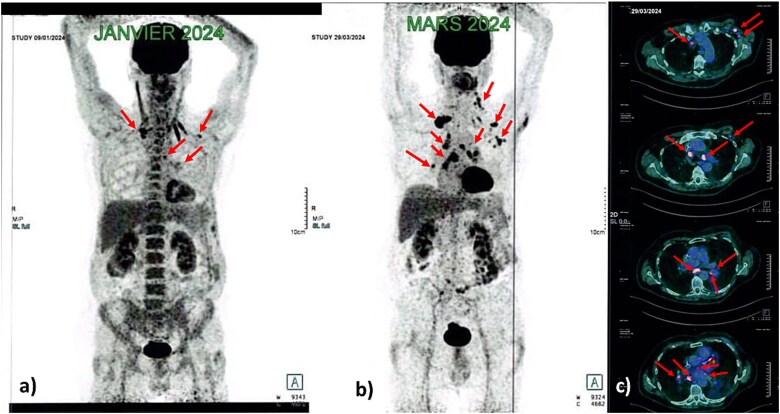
MIP view of FDG PET/CT from January 2024 (a) and march 2024 (b) showing metabolic progression of supradiaphragmatic nodal spread (arrows). Axial views of FDG PET/CT from march 2024 (c) showing hypermetabolic foci in the mediastinal and left axillary lymph nodes. MIP: Maximum intensity projection, FDG: Fluorodeoxyglucose, PET/CT: Positron emission tomography/computed tomography.
